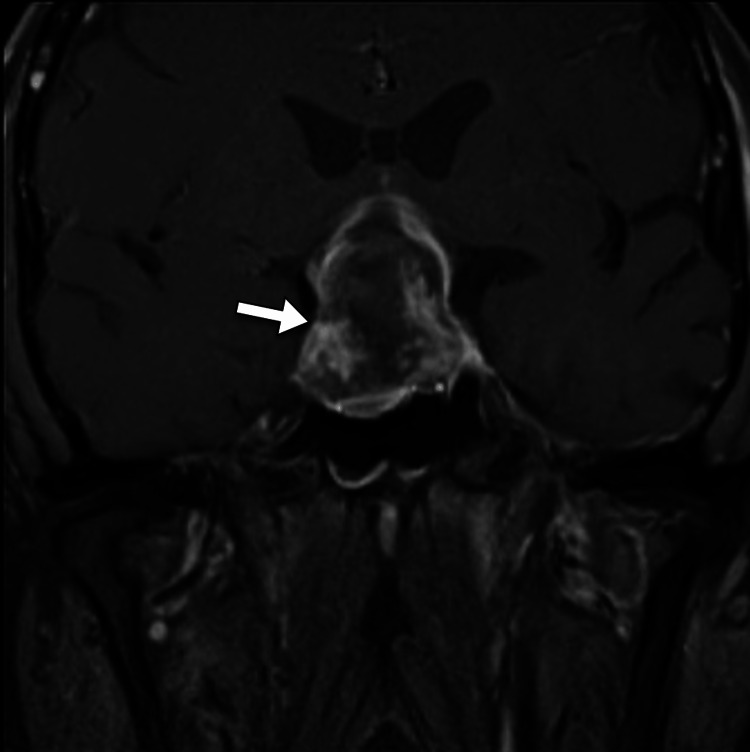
Coronal MRI post-contrast T1-weighted image shows a heterogeneously enhancing intrasellar mass (arrow) MRI: magnetic resonance imaging
